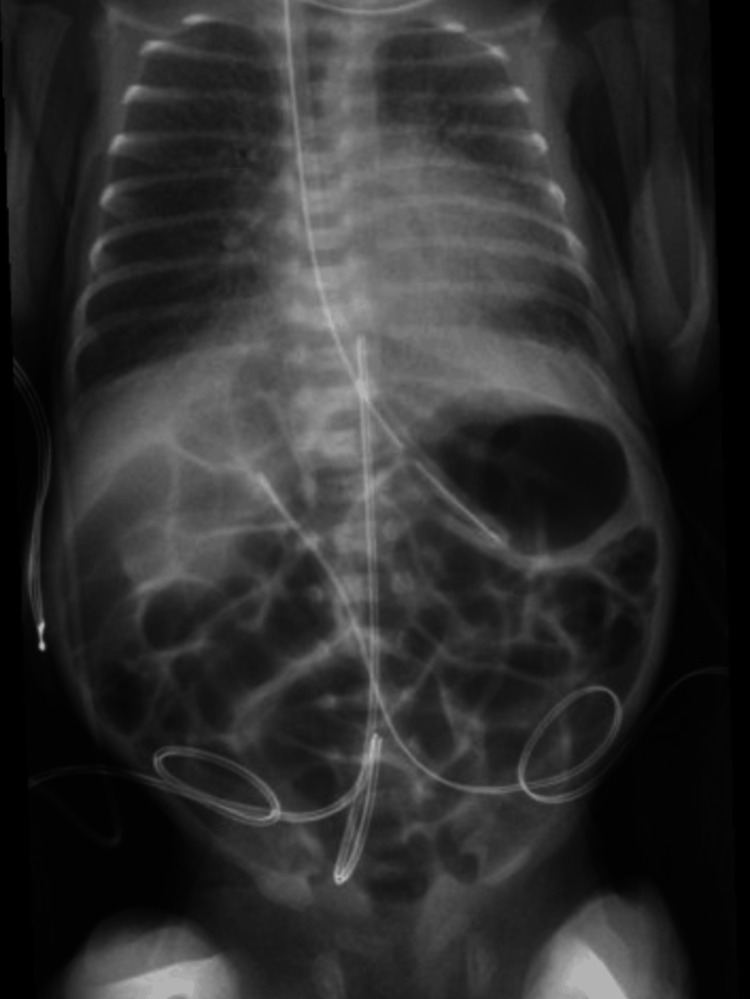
KUB radiograph showing gaseous distension of the stomach and small intestine without evidence of free intraperitoneal air or pneumatosis in the small intestinal wall KUB: kidney, ureter, and bladder
